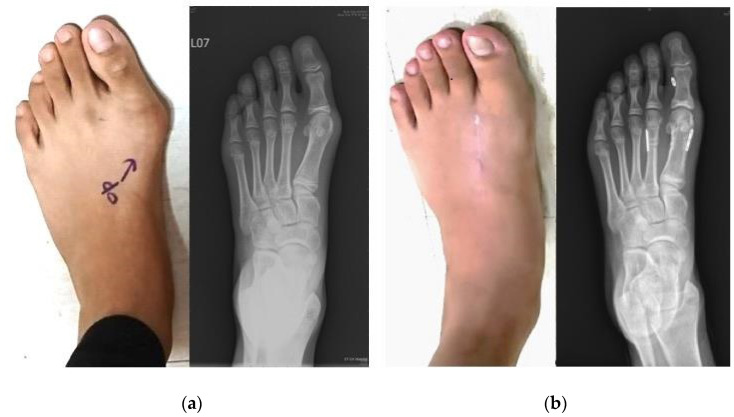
Clinical appearance and radiography (anteroposterior view) of the left foot. (a) Preoperative standing weight-bearing view and (b) 1-year follow-up postoperative standing weight-bearing view.
